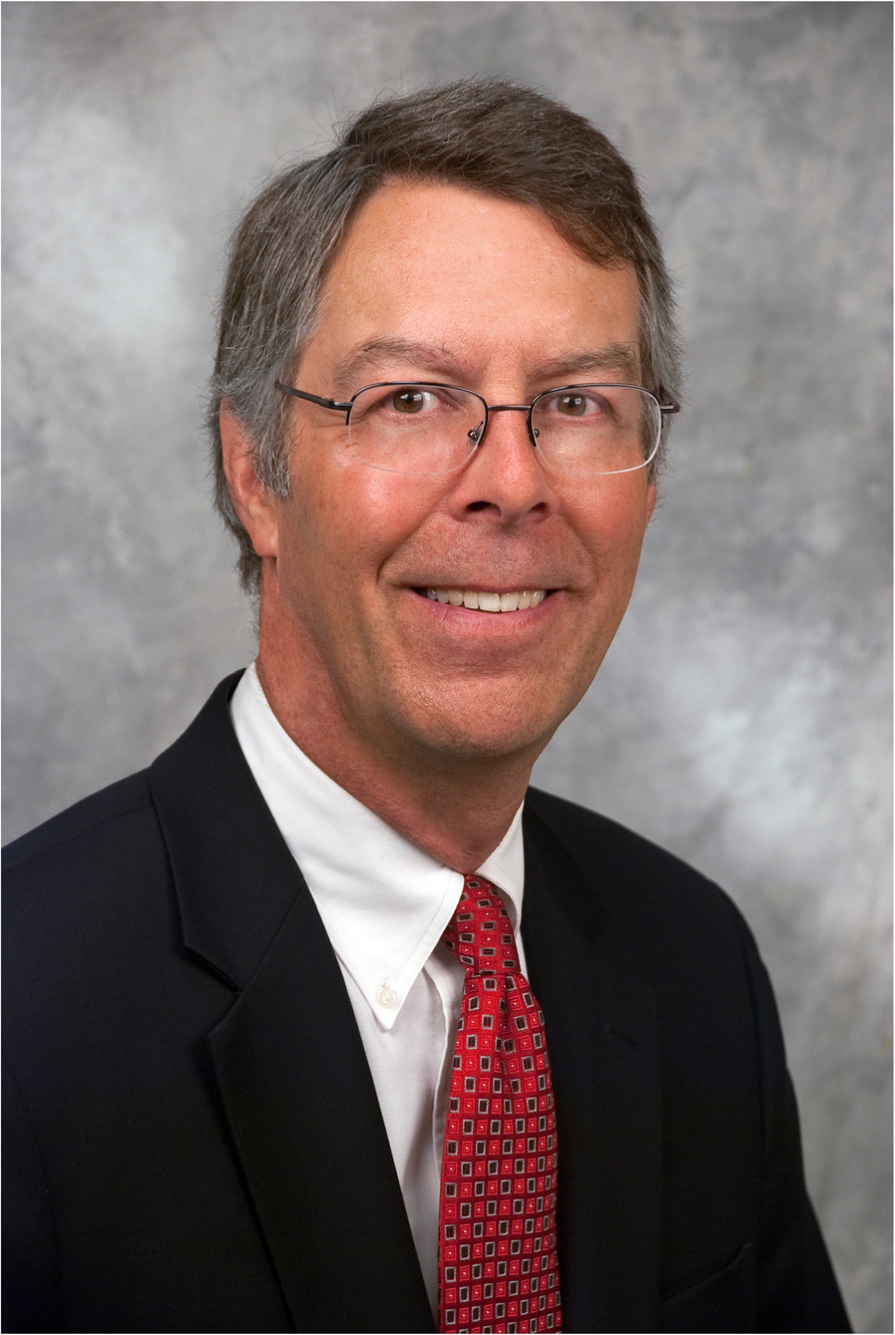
Peter C Albertsen is chief of urology and residency program director at the University of Connecticut Health Center in Farmington, CT. His research interests have focused on understanding the natural history of prostate cancer and the impact of treatment on health-related quality of life. He has published widely in the field of outcomes research in prostate cancer, including the impact of screening for prostate cancer. He is a past trustee of the American Board of Urology and has served as President of several national urological societies
